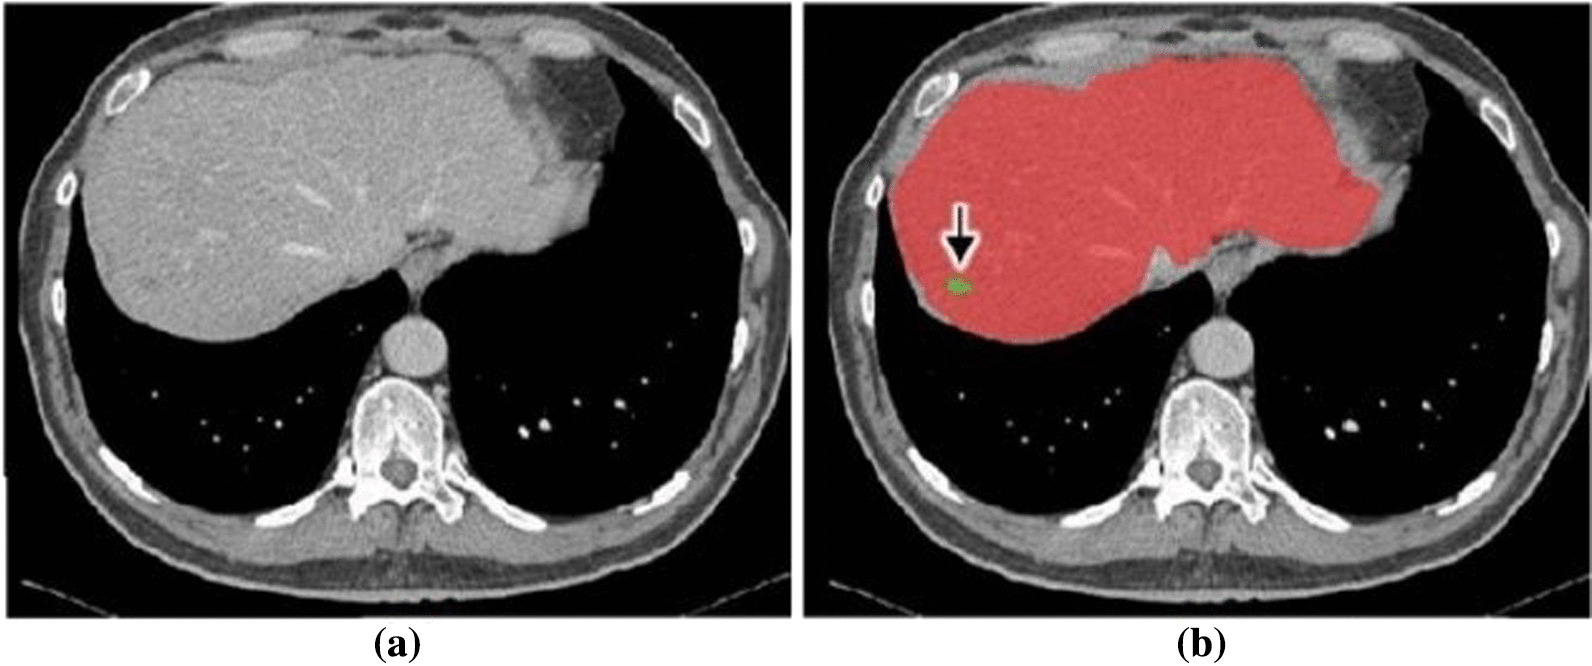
a Raw CT slice and b Segmented liver
